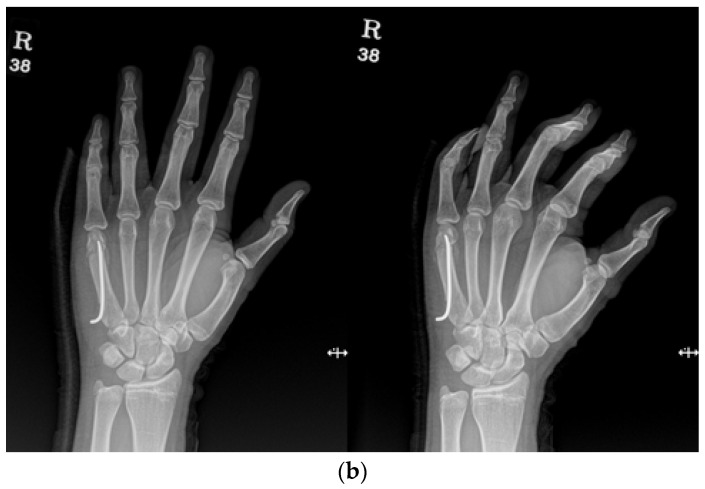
(a) Radiograph of a 16-year-old male patient with displaced little-finger metacarpal neck fracture. (b) Radiograph of a 16-year-old male patient who underwent the IP method using one intramedullary nail in the anterograde direction.
